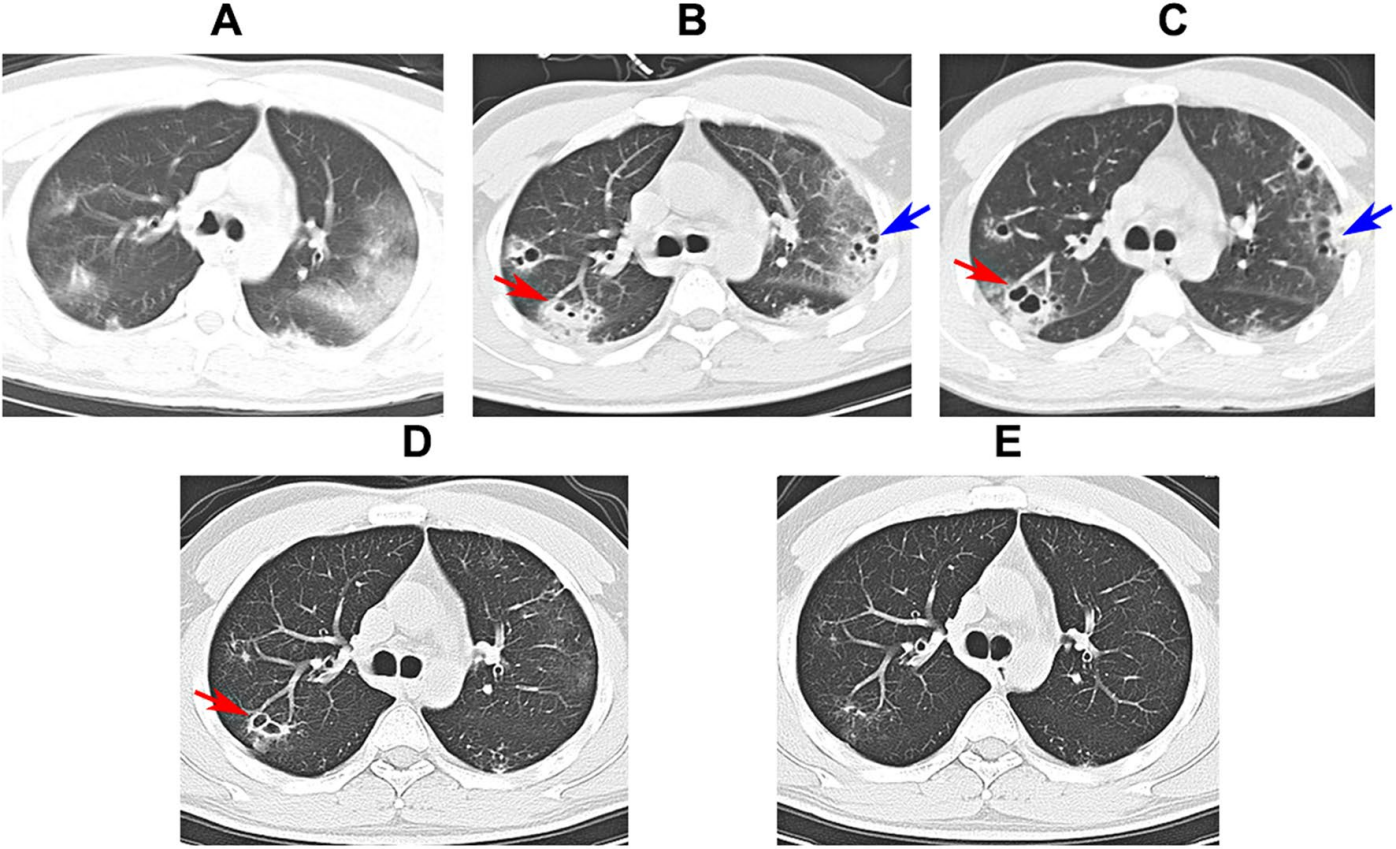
Chest computed tomography images. (A) Patchy consolidations in both lungs observed on the 1st day after admission. (B) Multifocal nodular opacities with new cavitations in left lung (blue arrow) and right lung (red arrow) observed on the 8th day after admission. (C) Residual cavitations in left lung (blue arrow) and enlarged cavitations in right lung (red arrow) observed on the 15th day after admission (discharge). (D) Resolved cavitations in left lung and resolved patchy consolidations with residual cavitations in right lung (red arrow) observed on the 4th day after discharge. (E) Resolved cavitations in both lungs observed 1 month later after discharge
